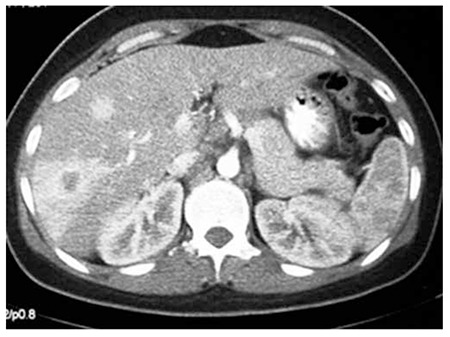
Computed tomography scan showing multiple hypodense regions in the right liver lobe
